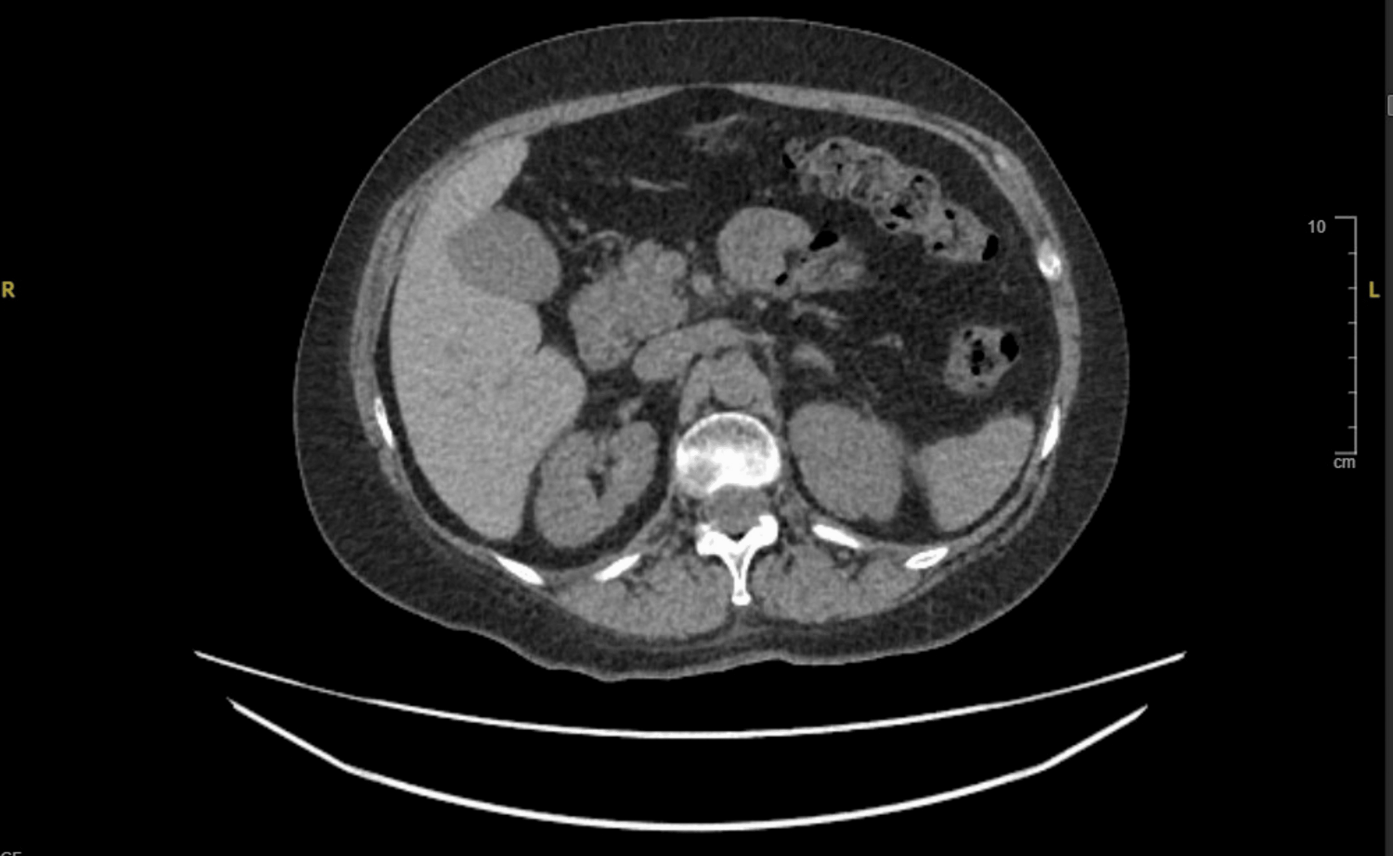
Computed tomography (CT) of the abdomen and pelvis, showing no evidence of adrenal hemorrhage.
